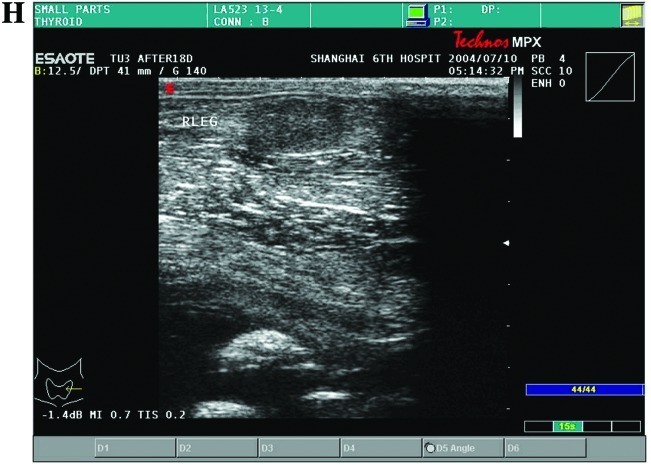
(A) The echo of rabbit leg muscle was homogeneous and hypoechoic, as detected by the two-dimensional ultrasound prior to HIFU radiation. (B) The echo of the HIFU lesion was hyperechoic at 10 min after HIFU radiation. (C) The echo at the center of the HIFU lesion became lower 1 h after HIFU radiation, there was a distinct hyperechoic border zone around the sonication lesions. (D and E) One to three days later, the echo of sonication lesions became lower. (F) One week later, there was a hypoechoic border zone surrounding the area of sonication. (G and H) Two to four weeks later, the volume of sonication lesions decreased gradually. The echo of sonication-injured tissue was homogeneous and hypoechoic.
